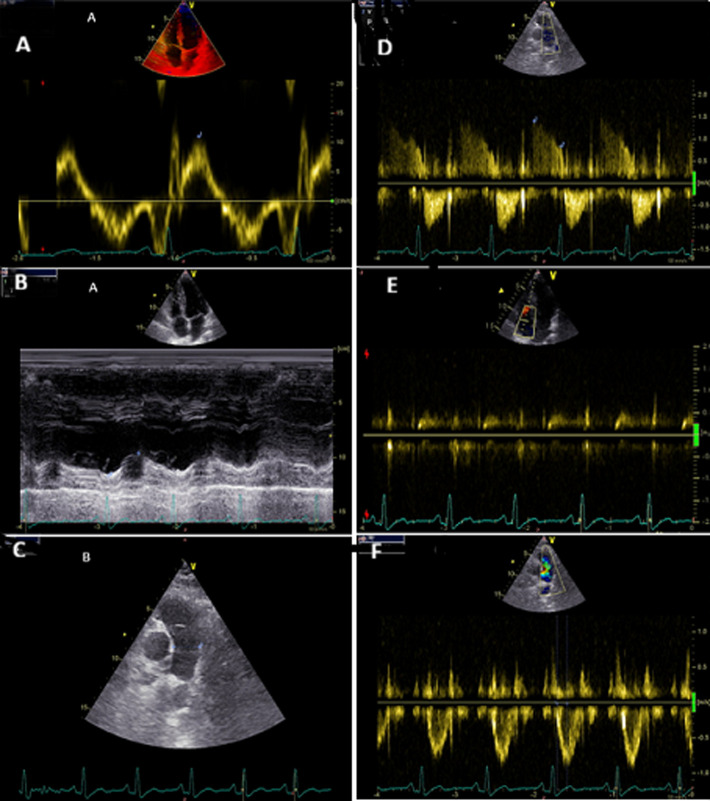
transthoracic echocardiography demonstrating normal right ventricular function without signs of pulmonary hypertension or right heart strain; A) right ventricular systolic function assessment: tissue Doppler imaging showing normal S wave velocity (0.11 m/s) and M-mode demonstrating normal TAPSE (1.9 cm); B) parasternal short-axis view showing normal pulmonary artery trunk diameter without evidence of thrombus; C) Doppler assessment of pulmonary pressures: pulmonary insufficiency flow; D) tricuspid regurgitation jet; E), and pulmonary artery acceleration time; F) demonstrating absence of pulmonary hypertension
